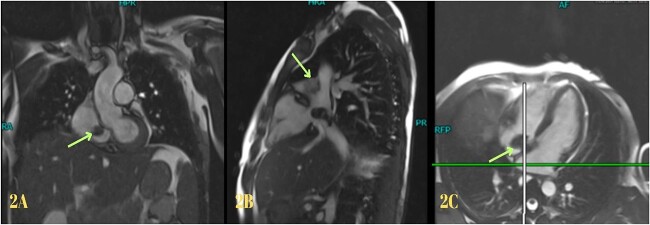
Cardiac magnetic resonance imaging showing myxoma.
